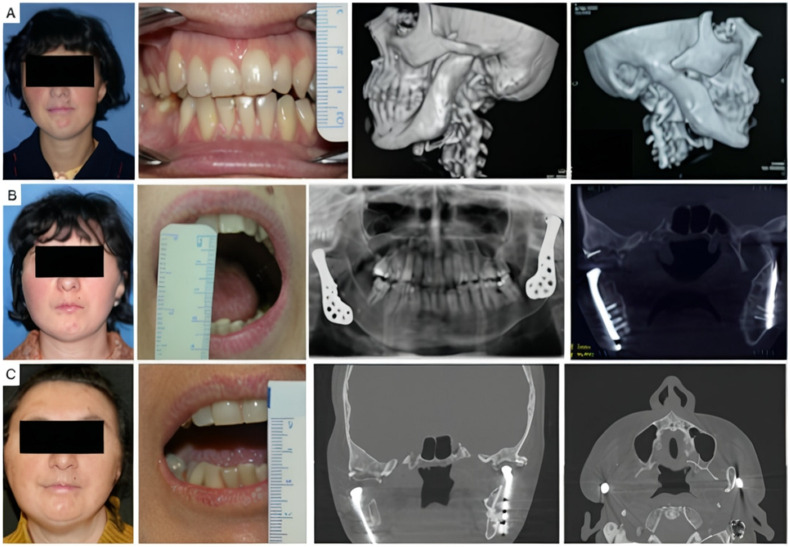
Clinical case of TMJ ankylosis caused by perinatal infection, treated by stock TMJ prostheses. (A) Clinical frontal preoperative view, preoperative maximum interincisal opening of patient (less than 1 cm) and preoperative 3D TC scan, that shows bilateral ankylosis. (B) Postoperative clinical view, maximum interincisal opening (3 cm) and postoperative radiographs of the patient at 2 years follow up. (C) Postoperative clinical view, maximum interincisal opening of patient (1.5 cm) and postoperative radiographs at 17 years follow up.
